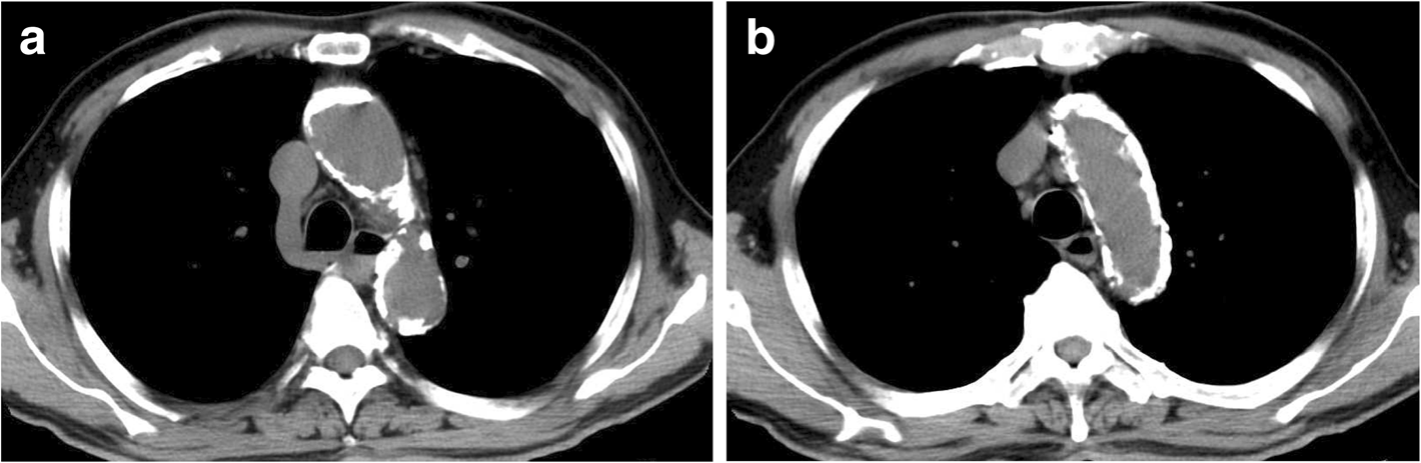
Computed tomography showing severe calcification of the ascending aorta (a) and aortic arch (b)
